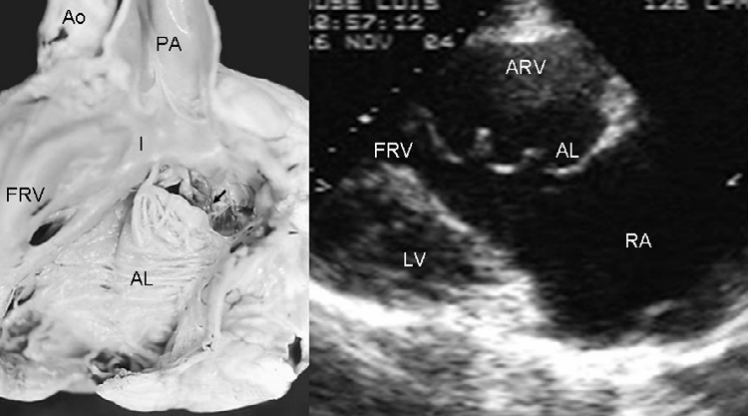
Internal view of the functional portion of the right ventricle shows a redundant anterior leaflet that inserts directly into the septum and right ventricular free wall, forming a curtain that separates the atrialized and functional portions of right ventricle. Note the functional tricuspid opening (arrow) in a subinfundibular position. The echocardiographic long axis image of right chambers shows the separation between the two portions of the right ventricle by a redundant and irregular anterior leaflet. PA: Pulmonary artery. Other abbreviations as before.
